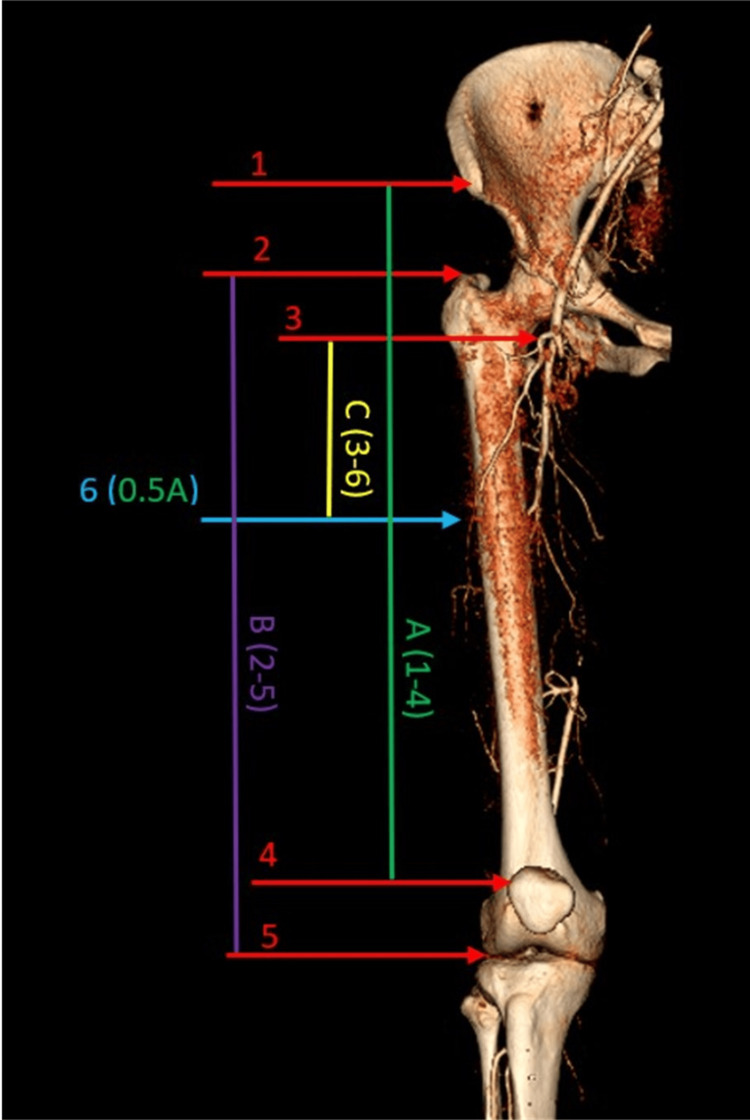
Measurements. Representation of the measurements taken in the study on a 3D computed tomography reconstruction. (1) the scan with ASIS, (2) the scan with the apex of the greater trochanter, (3) the scan with the origin of the descending branch of LFCA, (4) the scan with the superolateral corner of the patella, (5) the scan with the knee joint gap, (A) the distance between the scans (1) and (4), (6) the midpoint of the distance (A), (B) the distance between the scans (2) and (5), (C) the distance between the scan (3) and the midpoint (6). The anterior layers of the reconstruction are removed for better visualization of LFCA. ASIS: anterior superior iliac spine; LFCA: lateral femoral circumflex artery
